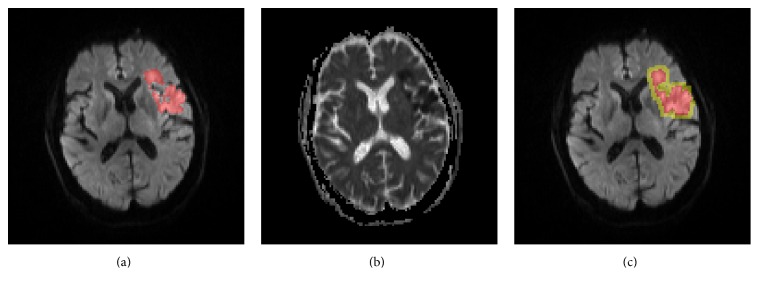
From (a) to (c), an axial slice of DWI with infarcts marked in red, the corresponding ADC, and the derived positive samples (in red) and negative samples (in yellow).
